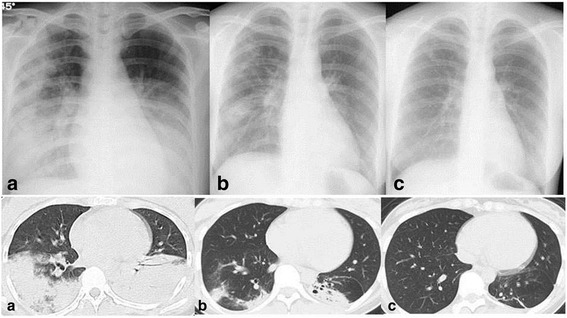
Chest X-ray showed bilateral infliltrates on admission (a upper). After starting PSL therapy on day 25, infiltrates were improved (b upper). Abnormal shadows on Chest X-ray disappeared 6 months after starting corticosteroid therapy (c upper). Chest CT showed consolidations on both lungs on admission (a lower) and the shadows were improved on day 25 after starting corticosteroid therapy (b lower). Six months after starting corticosteroid therapy, the consolidations disappeared (c lower)
